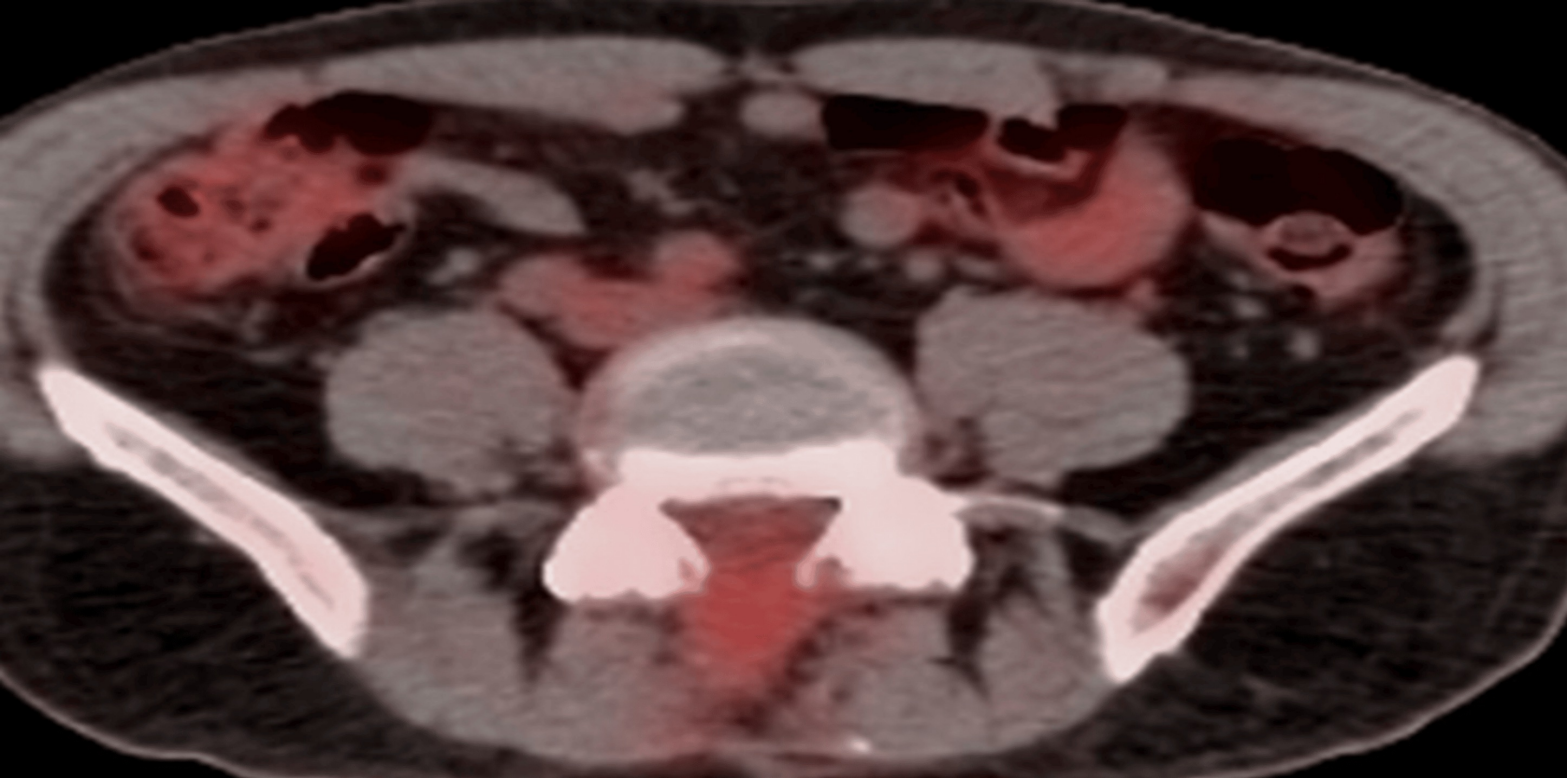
PET scan obtained September 11, 2023. Demonstrating no abnormal intrathecal fluorodeoxyglucose (FDG) uptake but increased FDG uptake in the laminectomy area is consistent with granulomatous tissue in the surgical bed.
